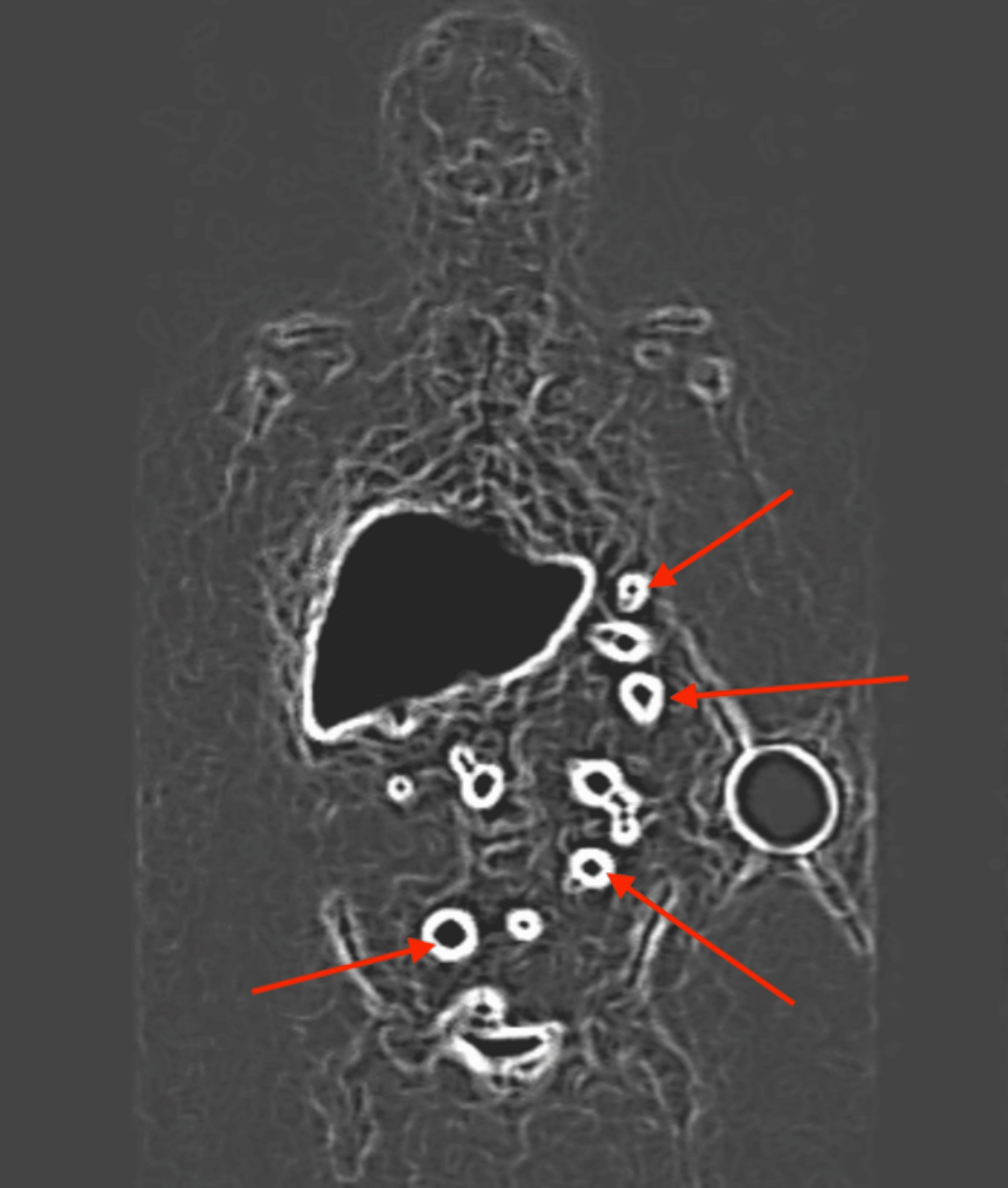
Tc-99m HDRBC scintigraphy Scintigraphic imaging demonstrates radiotracer uptake within multiple peritoneal nodules (red arrows), with activity equivalent to native splenic tissue, confirming the diagnosis of intra-abdominal splenosis. Tc-99m HDRBC: technetium-99m-labeled heat-damaged red blood cell
